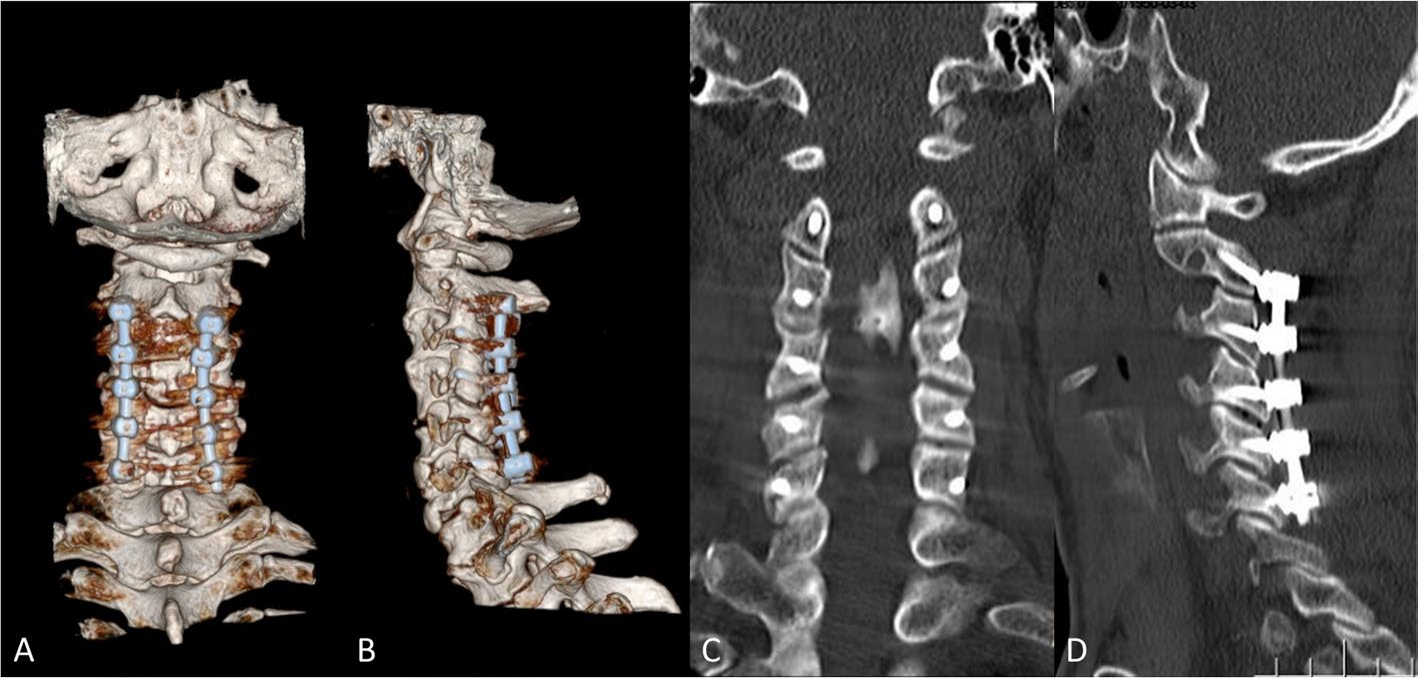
Three-dimensional surface renderings show typical trajectories of C2 pars screws from the dorsal (A) and right side (B) perspectives. CT scans show typical trajectories of C2 pars screws from the dorsal (C) and right side (D) perspectives
